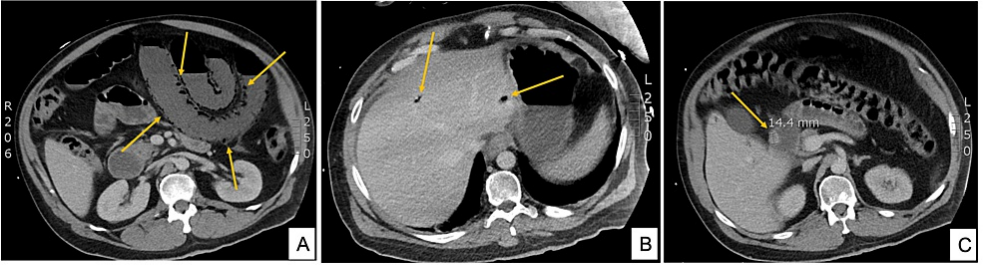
CT of patient two The images demonstrate extensive pneumatosis of the jejunum and ileum (A), portal vein gas (B), and an incidental 14 mm non-obstructing mural lesion within the first part of the duodenum (C) CT: computed tomography
